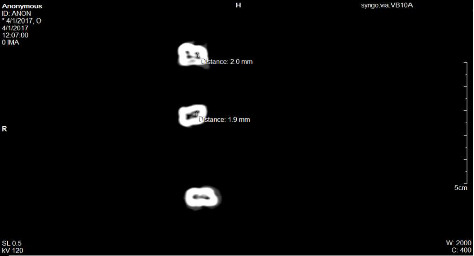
A CT slice at the level of the floor of the pulp chamber of teeth showing number of canal orifices and the distance between the canal orifices in a root of a tooth.
